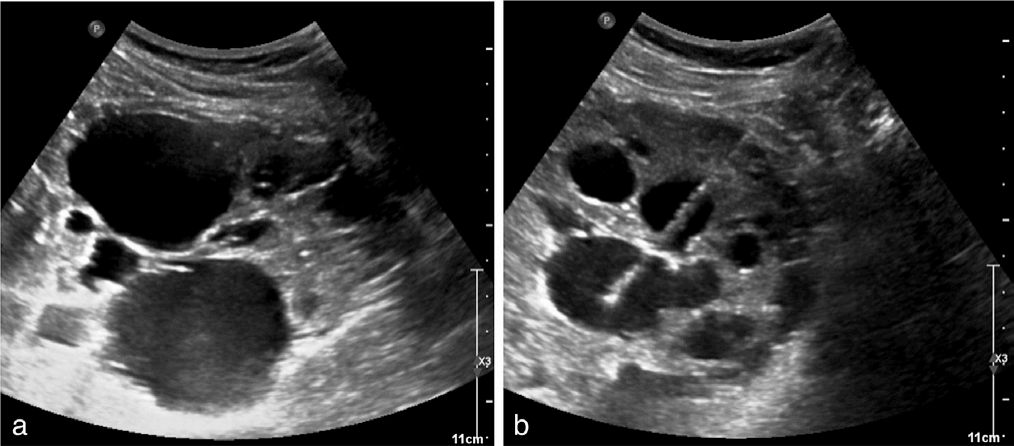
(a–b) A 48-year-old healthy female with history of ADPKD presents for routine outpatient renal cyst aspiration. Ultrasound of the right kidney (a) demonstrates multiple renal cysts. Renal cysts were aspirated under ultrasound guidance (b) using an 18-gauge Chiba needle. ADPKD, autosomal dominant polycystic kidney disease.
